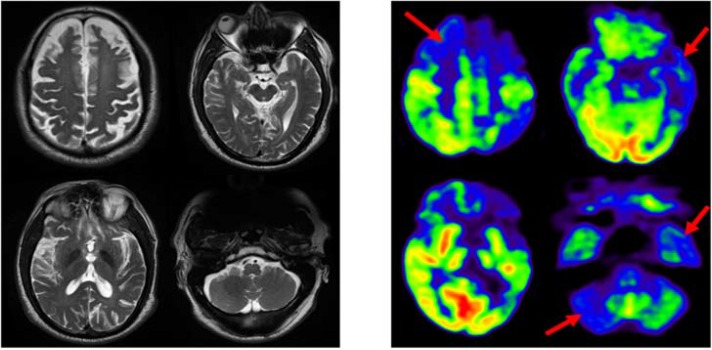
Fluorodeoxyglucose positron emission tomography/magnetic resonance imaging (FDG-PET/MRI) images of a 60-year-old man clinically diagnosed with frontotemporal dementia (FTD). MRI images are presented on the left side showing atrophy of the frontal, parietal, and temporal cortex. PET images are demonstrated on the right side showing decreased glucose metabolism in the bilateral frontal cortex, anterior cingulate cortex, left parietotemporal cortex, lateral temporal cortex, superior temporal cortex, medial temporal cortex, and right cerebellum.
